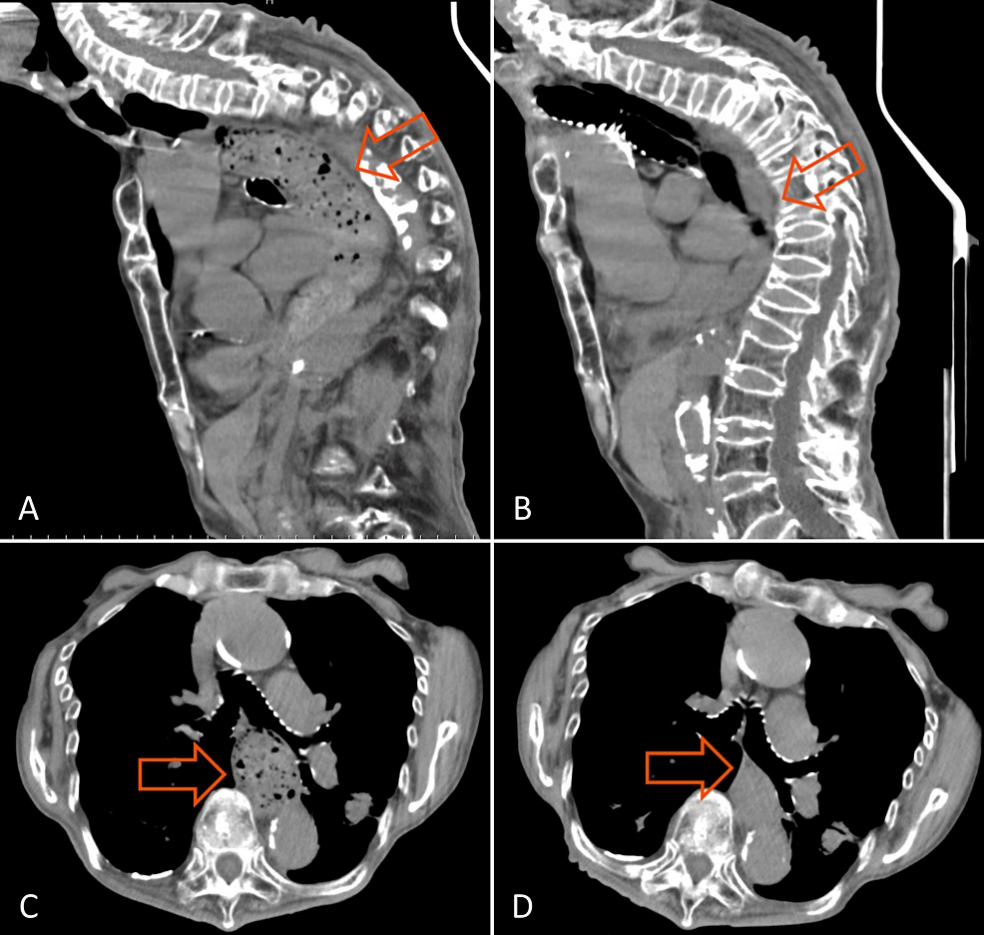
CT taken at chest pain (A, C): (A) Sagittal section showing the food mass filled the lower to upper thoracic esophagus. (C) Horizontal section at the level of the bronchial bifurcation showing the esophagus also filled with a food bolus. CT taken three hours after chest pain (B, D): (B) Sagittal section revealing most of the food mass had passed through but remained partially in the esophagus. (D) Small amount of liquid was observed in the horizontal section at the level of the bronchial bifurcation
